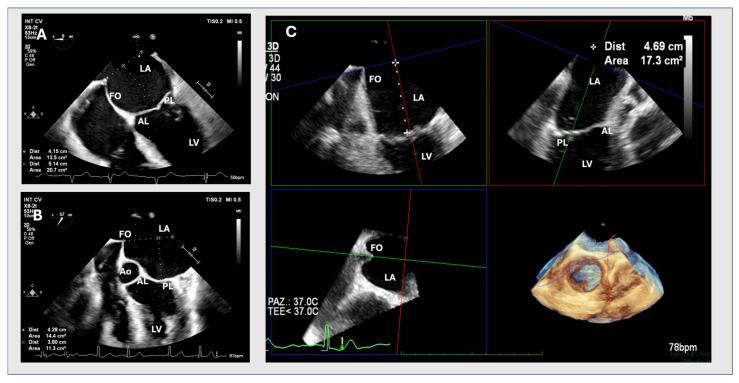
Figures (A,B) show an optimal 2D and suboptimal upper transoesophageal view, respectively. Figure (C) shows a multiplanar three-dimensional reconstruction. AL: anterior leaflet; Ao: aortic valve, FO: fossa ovalis; LA: left atrium; LV: left ventricle; PL: posterior leaflet.
